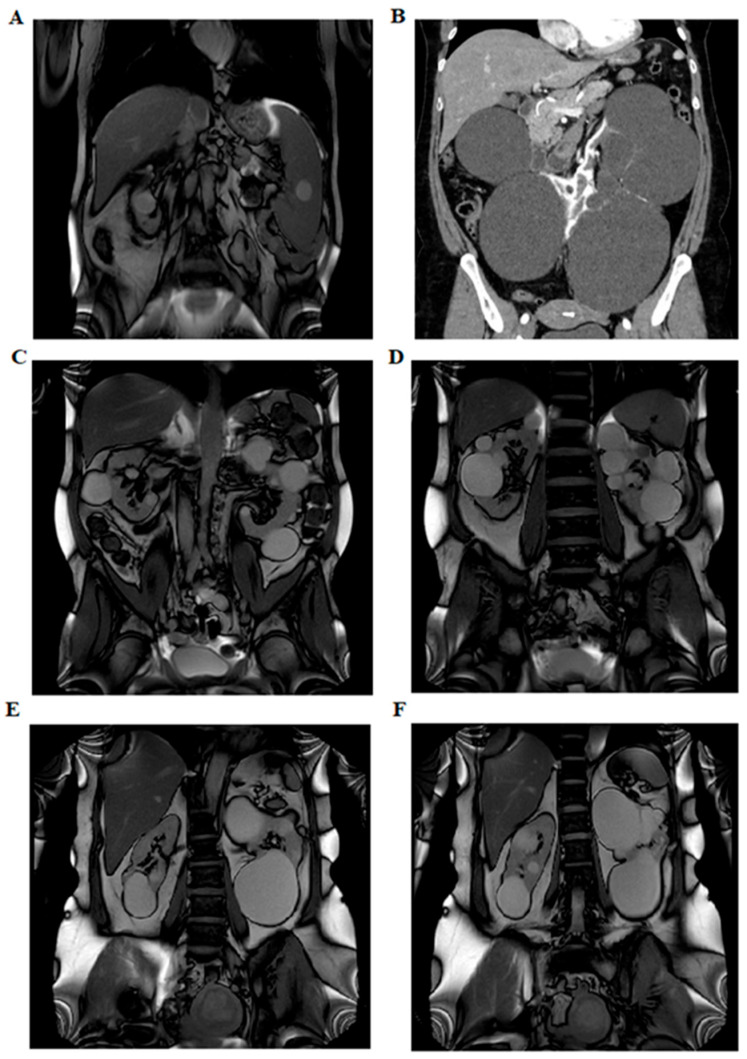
Abdominal imaging performed with nuclear magnetic resonance imaging and computed axial tomography. Panel (A) shows left ptosed kidney in left lumbar iliac spine with extra-rotated pelvis and multiple bilateral renal cysts (BO-183). Panel (B) shows several bilateral cortical renal cysts (B0-214). The panels (C,D) show multiple bilateral kidney cysts and exophytic cysts (BO-213). The panels (E,F) show few large renal cysts and no hepatic cysts (BO-216).
